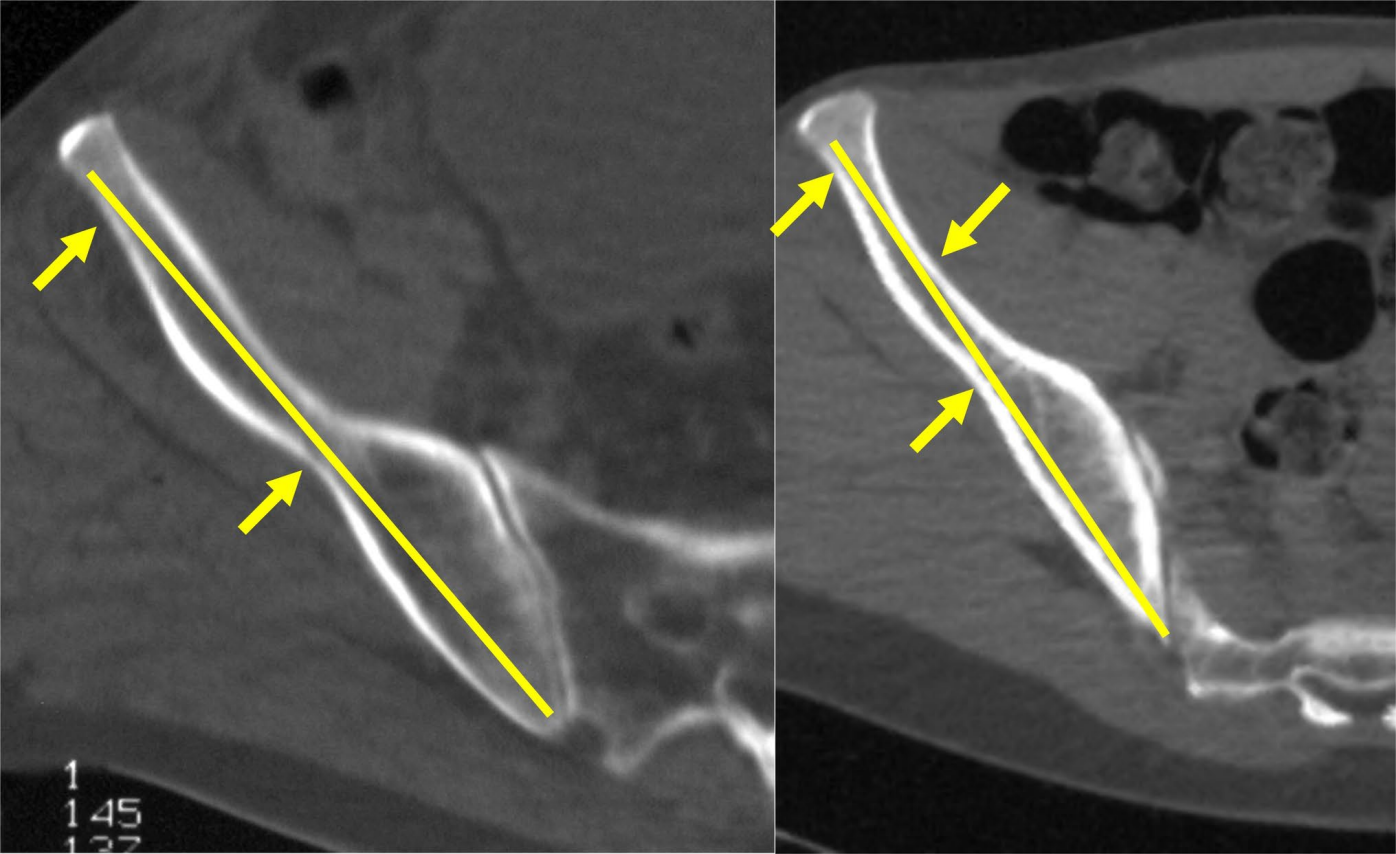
Narrow zones of the supraacetabular corridor (SAC) in axial CT-scans
